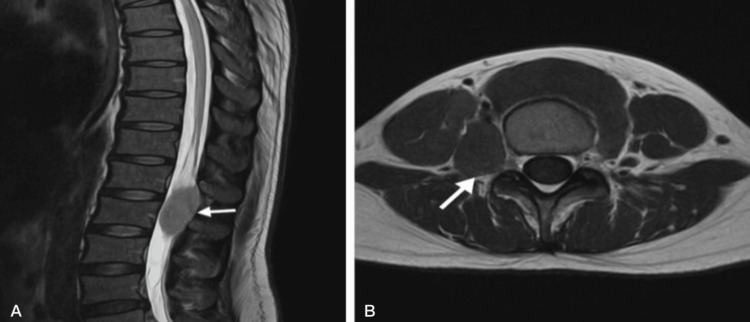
Sagittal and axial MRI of the lumbar spine showing a left paraspinal mass consistent with extramedullary hematopoiesis (A) Sagittal T2-weighted MRI of the lumbar spine shows a well-defined paraspinal soft tissue mass at the L4–L5 level (white arrow), causing mild compression of the dural sac and anterior displacement of the spinal cord. (B) Axial T2-weighted MRI confirms the presence of a left paraspinal mass that is isointense to mildly hyperintense relative to adjacent muscle (white arrow), without evidence of vertebral destruction or spinal canal invasion. These imaging features are consistent with extramedullary hematopoiesis in a patient with transfusion-dependent beta-thalassemia.
